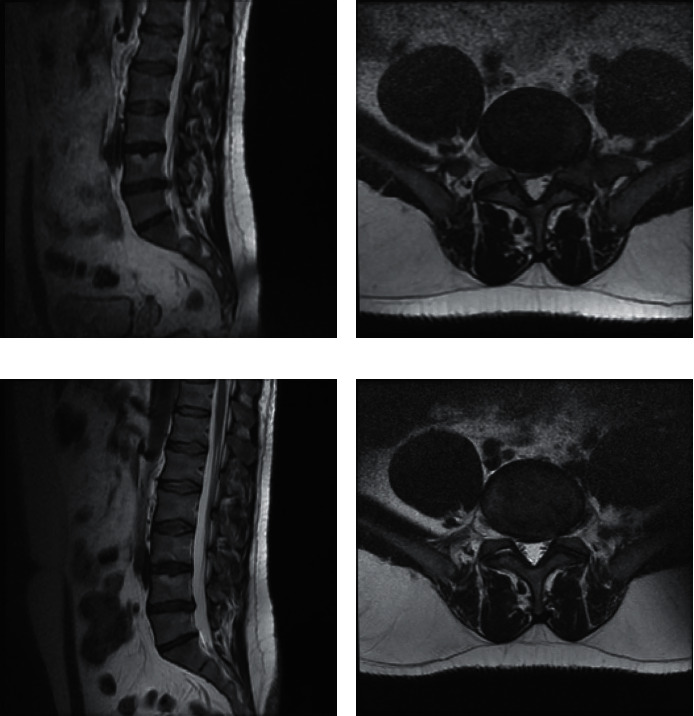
Preoperatively and postoperatively axial and sagittal images of MRI before/after Holmium-YAG laser-assisted ELND. (a) Preoperative sagittal MRI image of the patient. (b) Preoperative axial MRI image of the patient. (c) Postoperative sagittal MRI image of the patient. (d) Postoperative axial MRI image of the patient.
